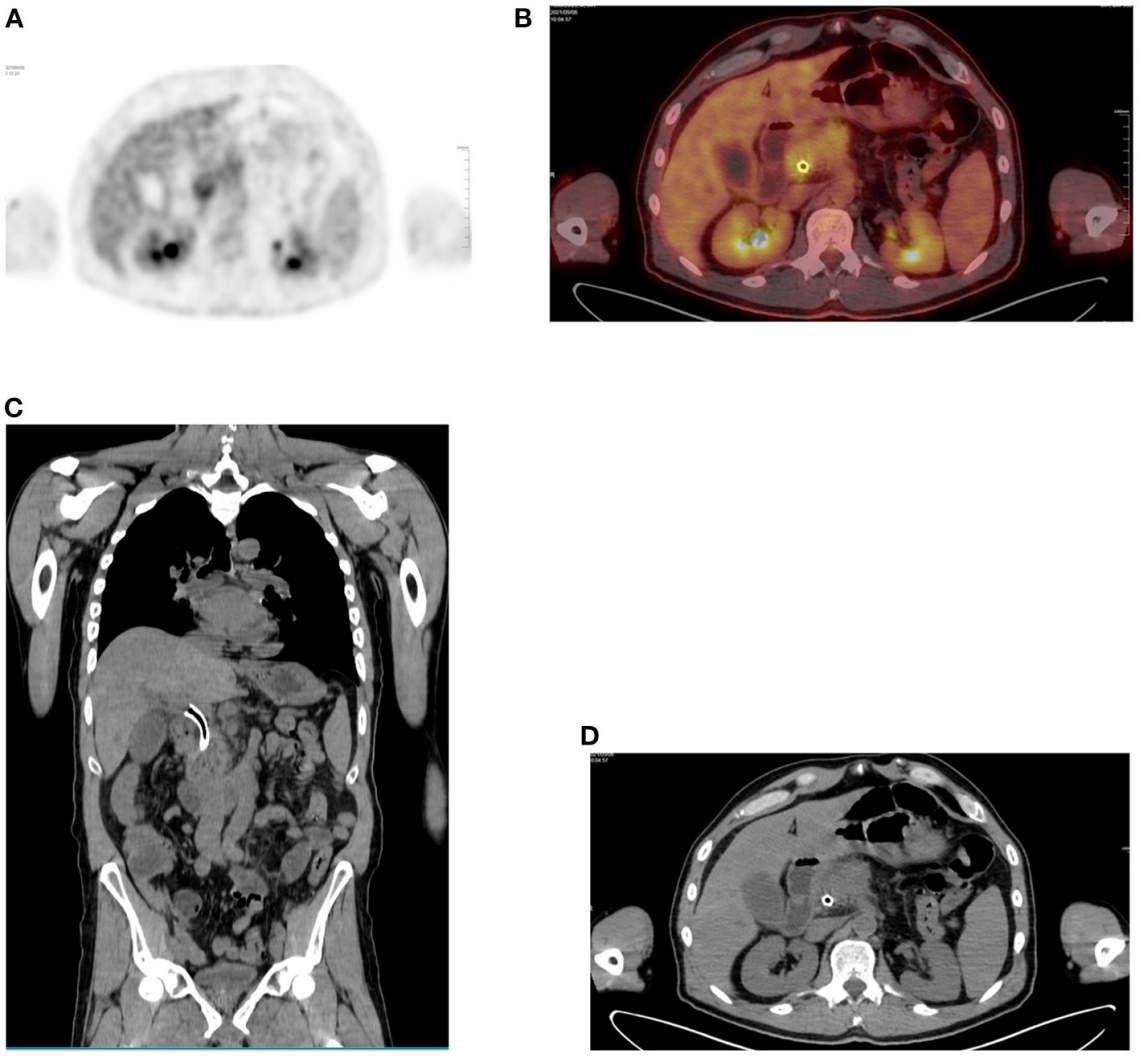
Focal uptake due to stent. (A) FDG PET Axial and (B) combined PET/CT with focal liver uptake in a 64-year-old male patient with pancreas adenocarcinoma and who underwent a placement of stent at CBD. Corresponding anatomical images alone, both coronal and axial planes (C,D) show the stent in place.
